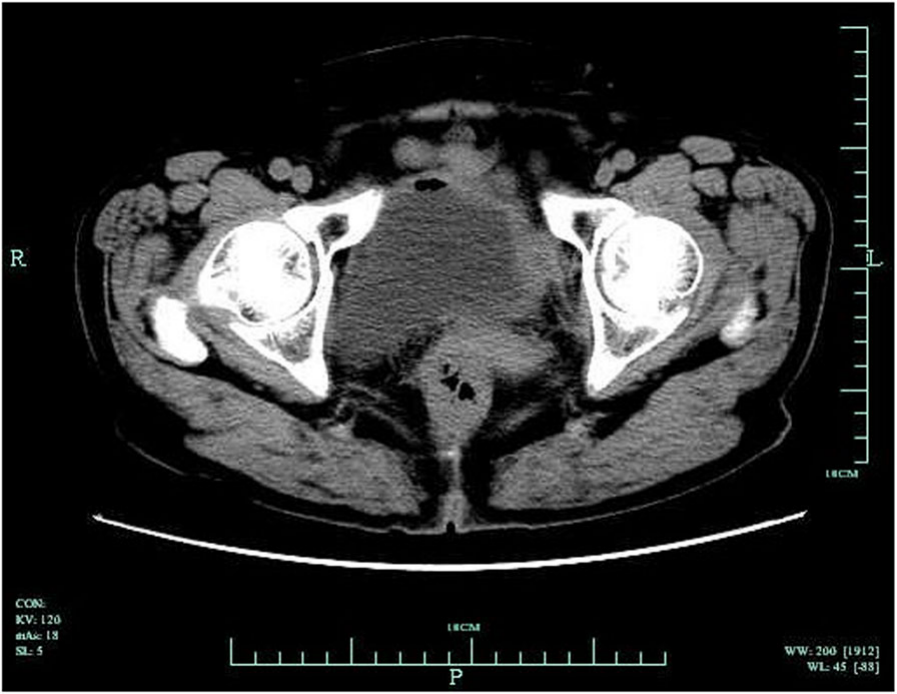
Computed tomography showing the thickened local left bladder wall and the internal iliac muscle
